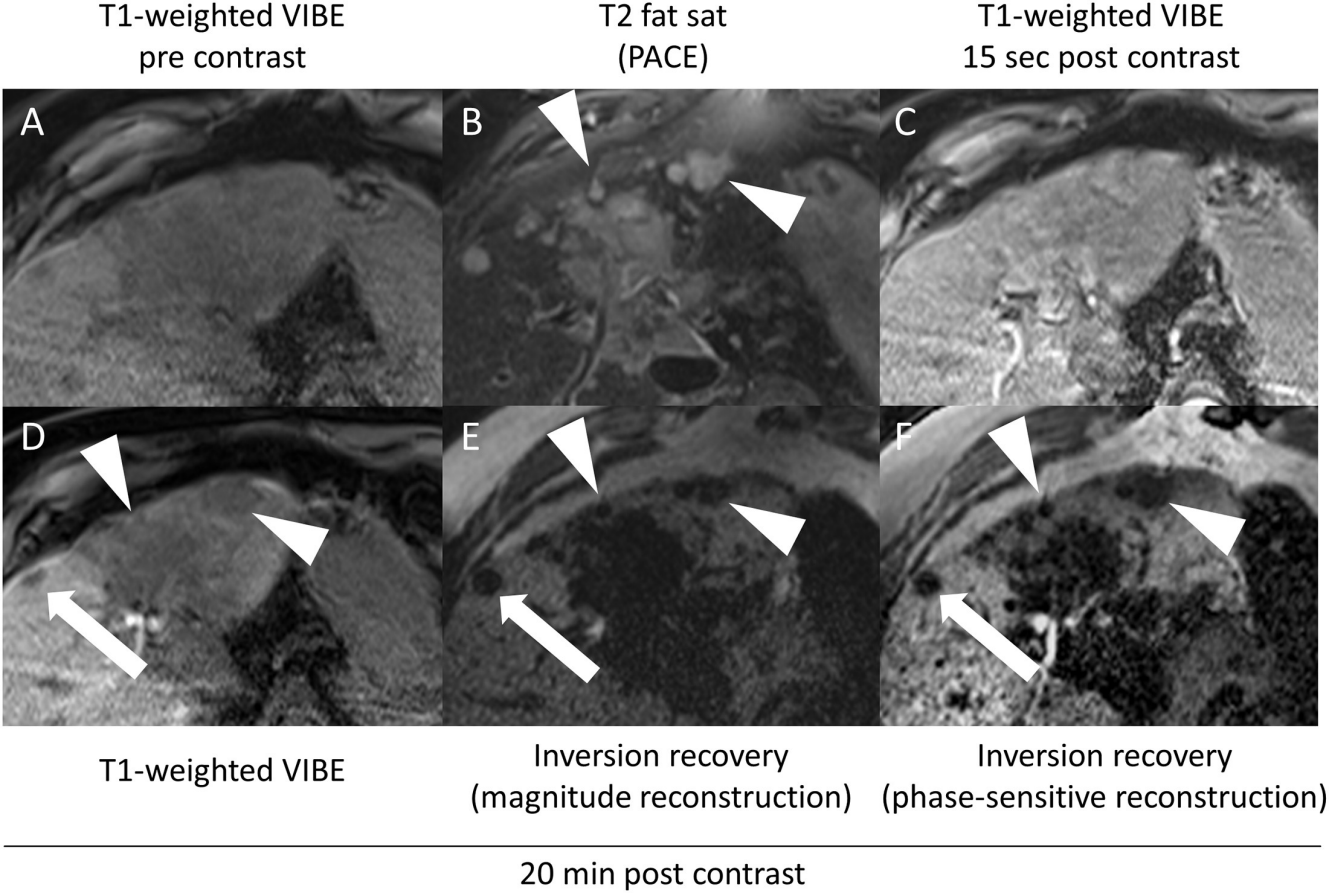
Metastatic tumour in an 80-year-old man with central cholangiocellular carcinoma with metastatic spread. Transverse T1-weighted VIBE (A), and T2 TSE-weighted (B) images. Dynamic VIBE imaging started 15 seconds after intravenous administration of gadoxetate disodium (C). (D) shows VIBE images 20 minutes after contrast agent administration, (E) inversion-recovery with magnitude reconstruction and with phase-sensitive reconstruction (F). The subcapsular lesion is more clearly depicted (white arrow) than on conventional VIBE images. Additionally, several lesions within the liver parenchyma affected by long-standing cholestatic disease can be clearly delineated on inversion recovery images, whereas reduced gadoxetate disodium uptake diminished delineation on conventional VIBE images (arrowheads).
